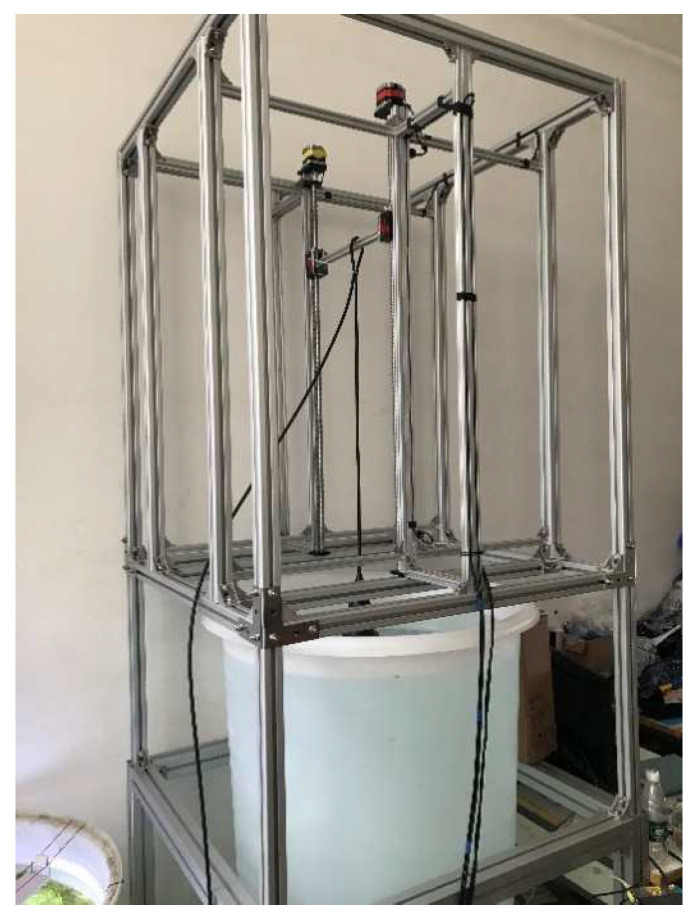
Longitudinal-ultrasonic-transducer error-calibration-test platform with a height of 1500 mm.
